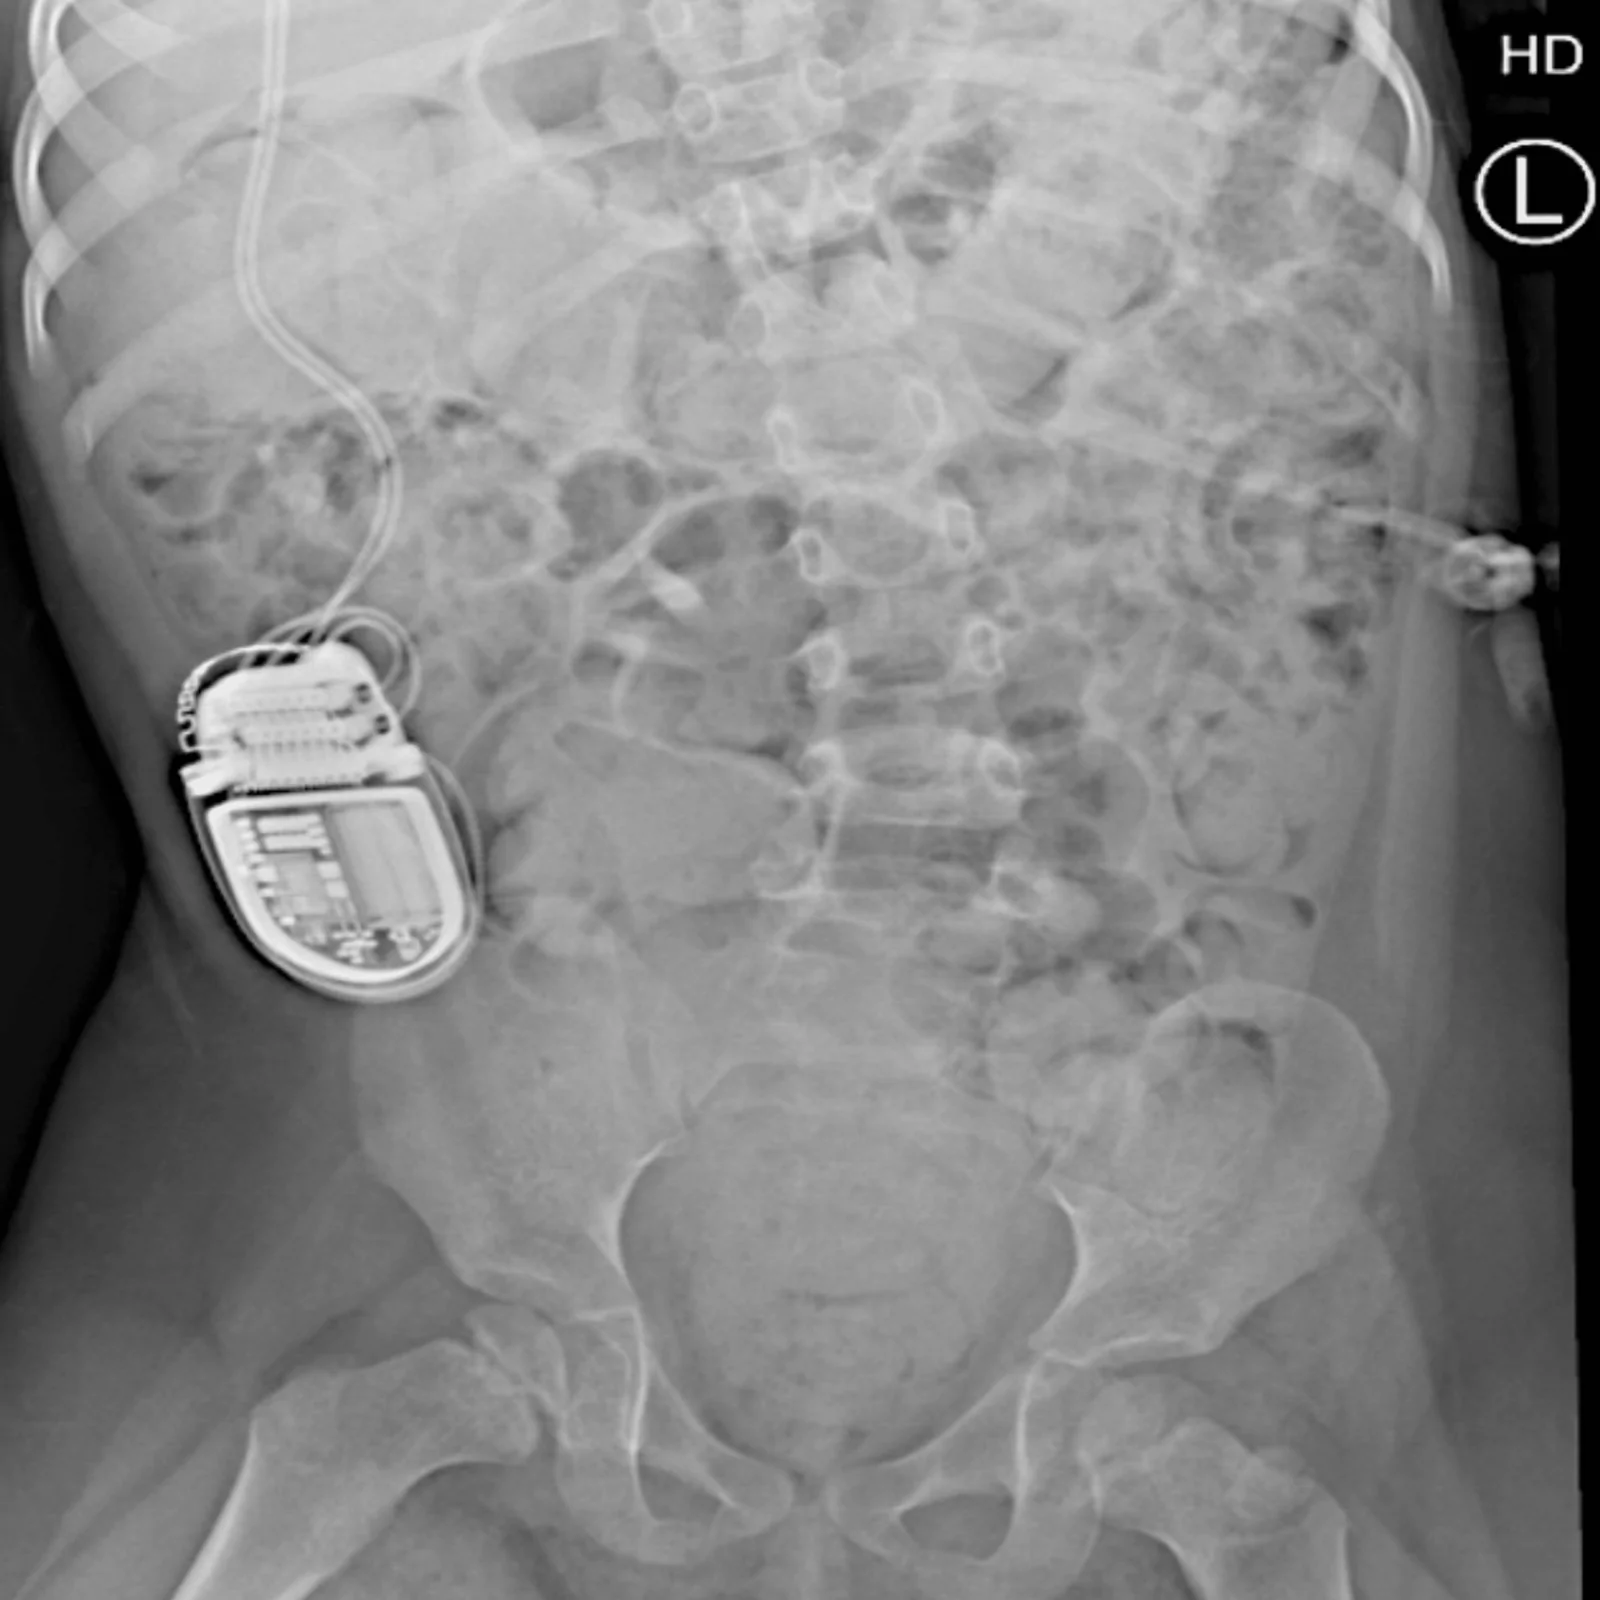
X-ray abdomen Day 4 post admission: small amount of persistent pneumatosis in the right flank; gastrostomy and deep brain stimulator noted
